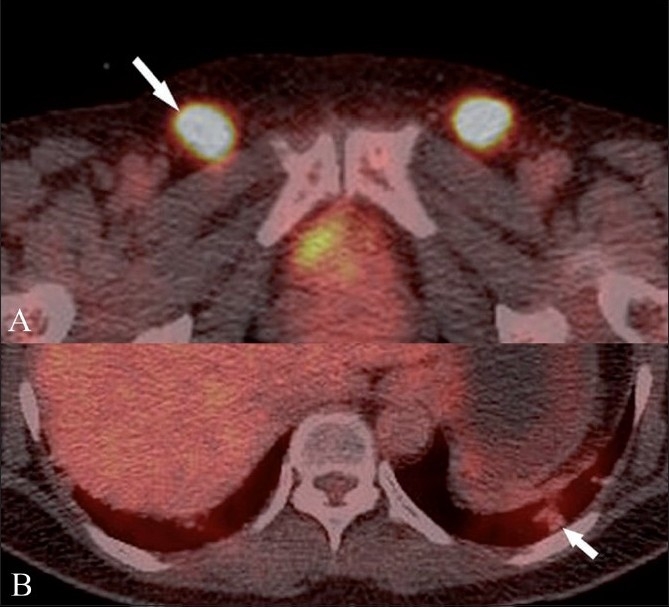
FDG PET/CT shows increased uptake in the inguinal lymph nodes (arrow in A) with no significant uptake in the lung nodules (arrow in B)
